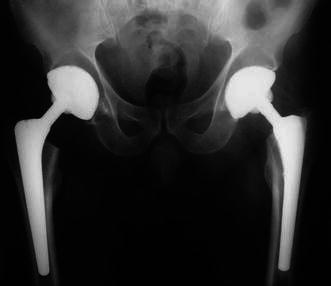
Radiograph of bilateral THA at follow-up: anatomic stem on the *left* side and right straight stem on the *right* side
